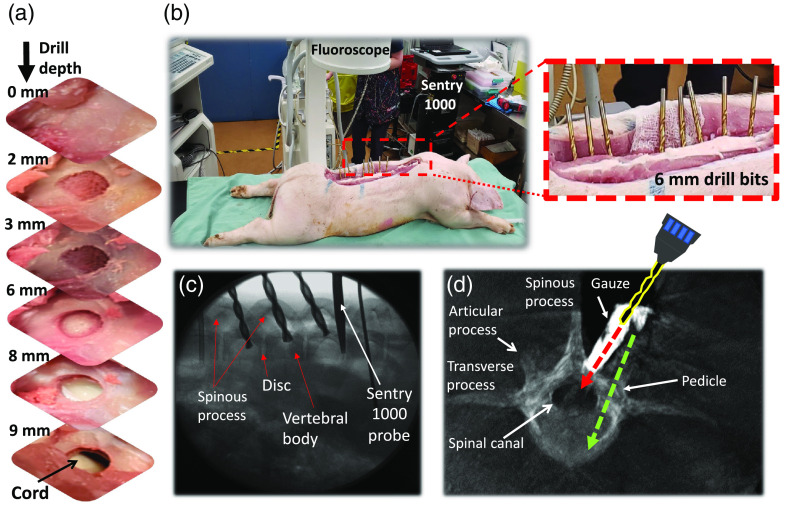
(a)–(c) *In situ* and (d) *in vivo* drilling and RS procedures. (a) Photographs at each stage of drilling demonstrating mm-level depth control and showing the bone surface and the drilling cavity at the cortical/trabecular interface (2 mm), trabecular bone (3 mm), trabecular/cortical interface (6 mm), epidural space (8 mm), and spinal cord breach (9 mm). (b) *In situ* drilling under fluoroscopy imaging guidance with a labeled radiograph shown in (c). (d) 3D CT image guidance in the axial view showing labeled vertebra with mis-directed drill direction toward spinal canal (red arrow), indicated by insertion of contrast-infused gauze. The green arrow indicates the correct drill trajectory through the pedicle.
